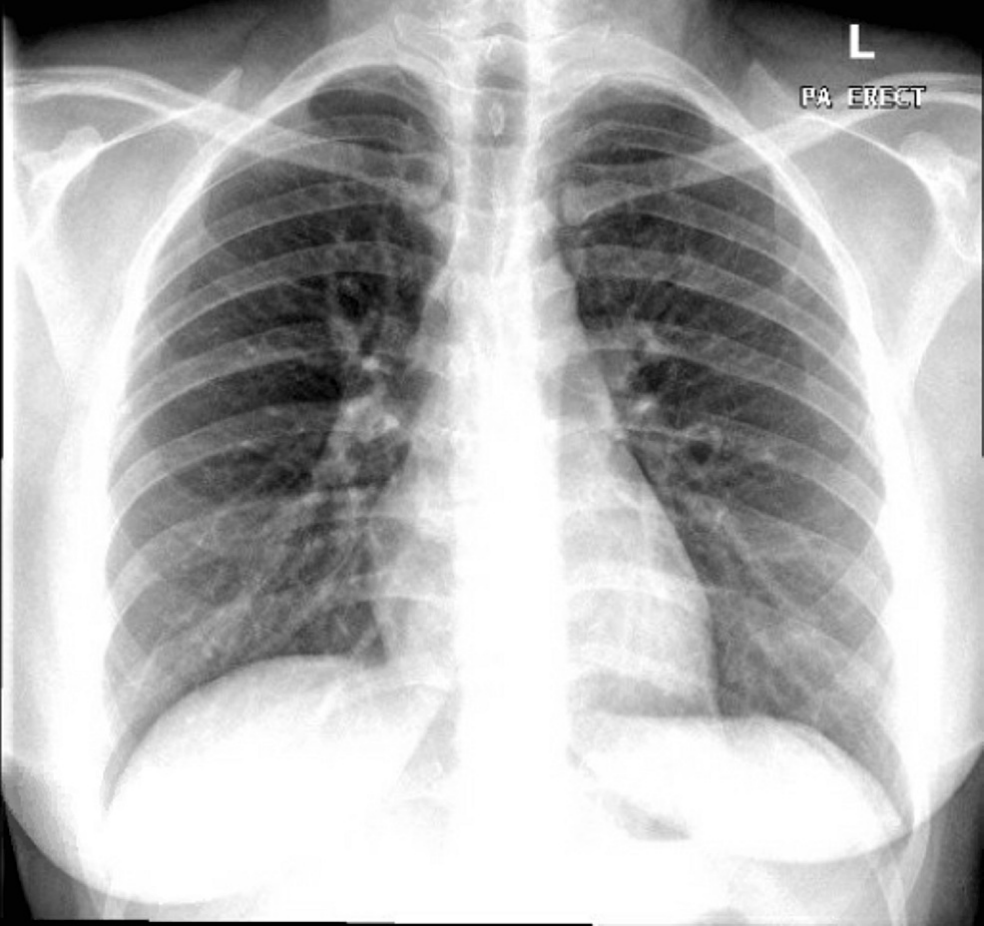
Chest x-ray at presentation.
